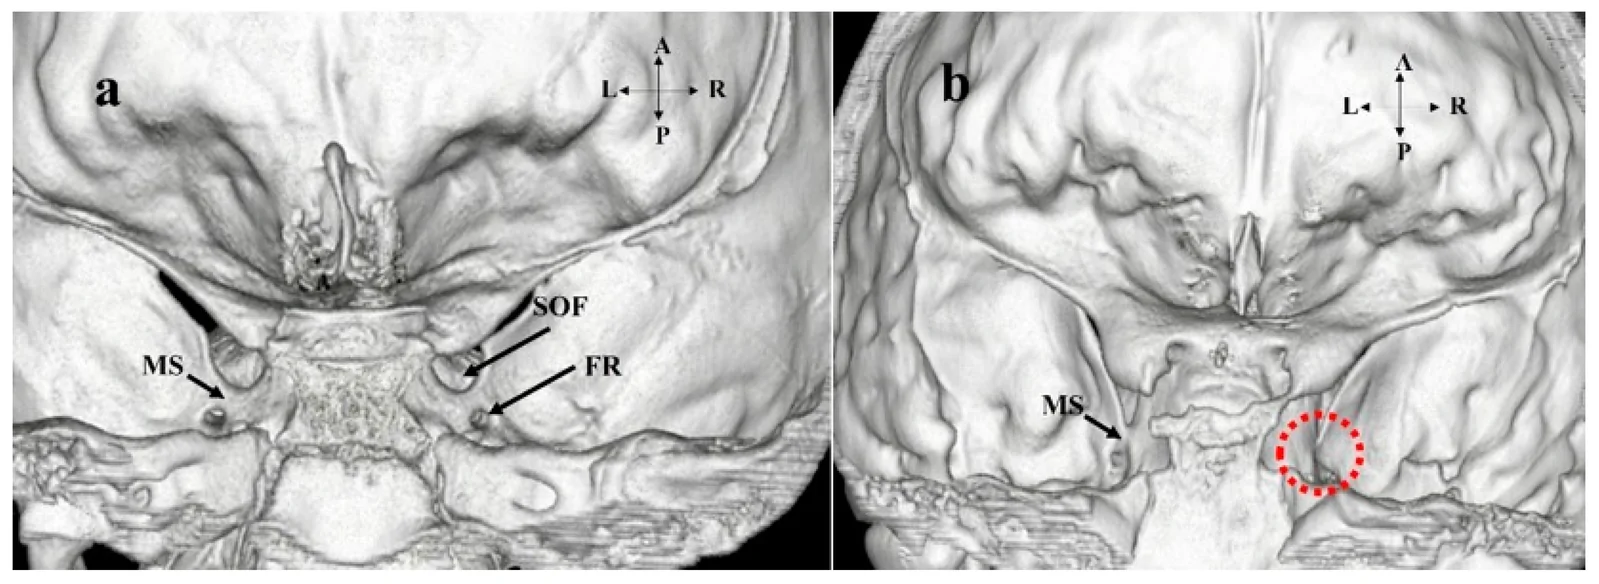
Three-dimensional computed tomography reconstructions demonstrating (a) bilateral maxillary struts (MS) between the foramen rotundum (FR) and superior orbital fissure (SOF) and (b) a rudimentary right-sided MS (red dashed circle). A, anterior; P, posterior; R, right; L, left.
